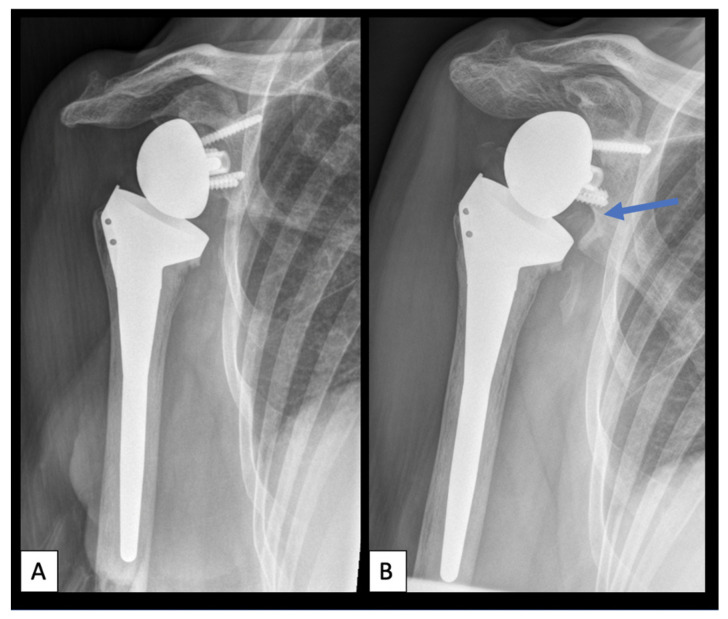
Scapular notching. (A): Anteroposterior radiograph of a right shoulder one-year postoperatively. (B): Anteroposterior radiograph of a right shoulder eight years postoperatively with scapular notching grade three according to the classification of Sirveaux [10]. Blue arrow demonstrating the area of scapular notching at the inferior glenoid.
